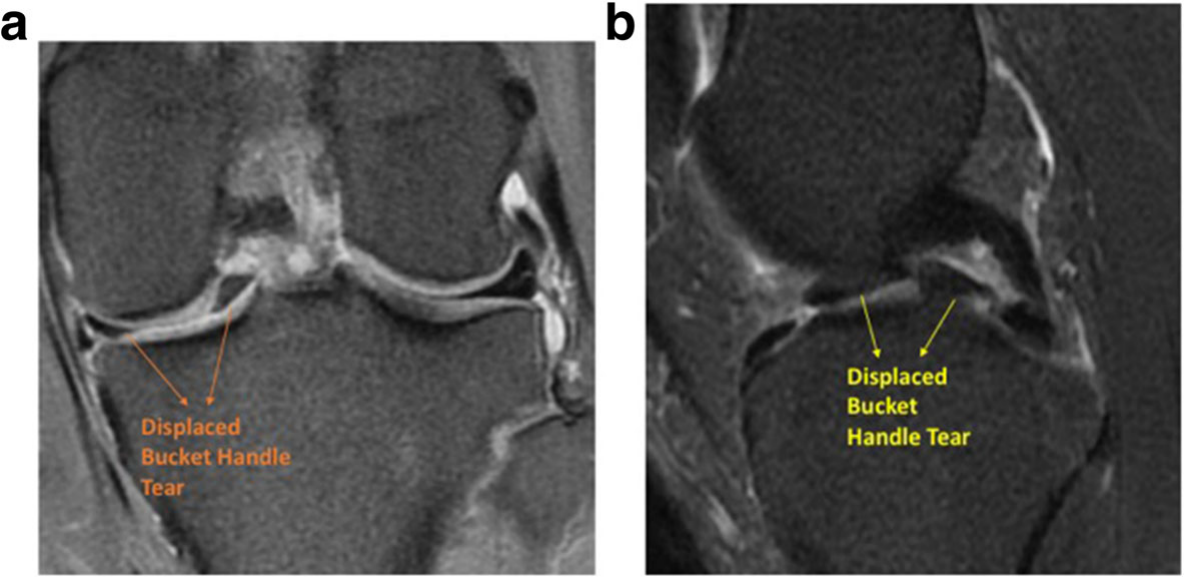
Magnetic resonance imaging of the visualized meniscus tear. a Coronal proton density fat suppression image of the patient’s left knee showing a displaced bucket-handle tear of the medial meniscus. b Sagittal T2 fat suppression image of the patient’s left knee again showing the displaced medial meniscus bucket-handle tear
